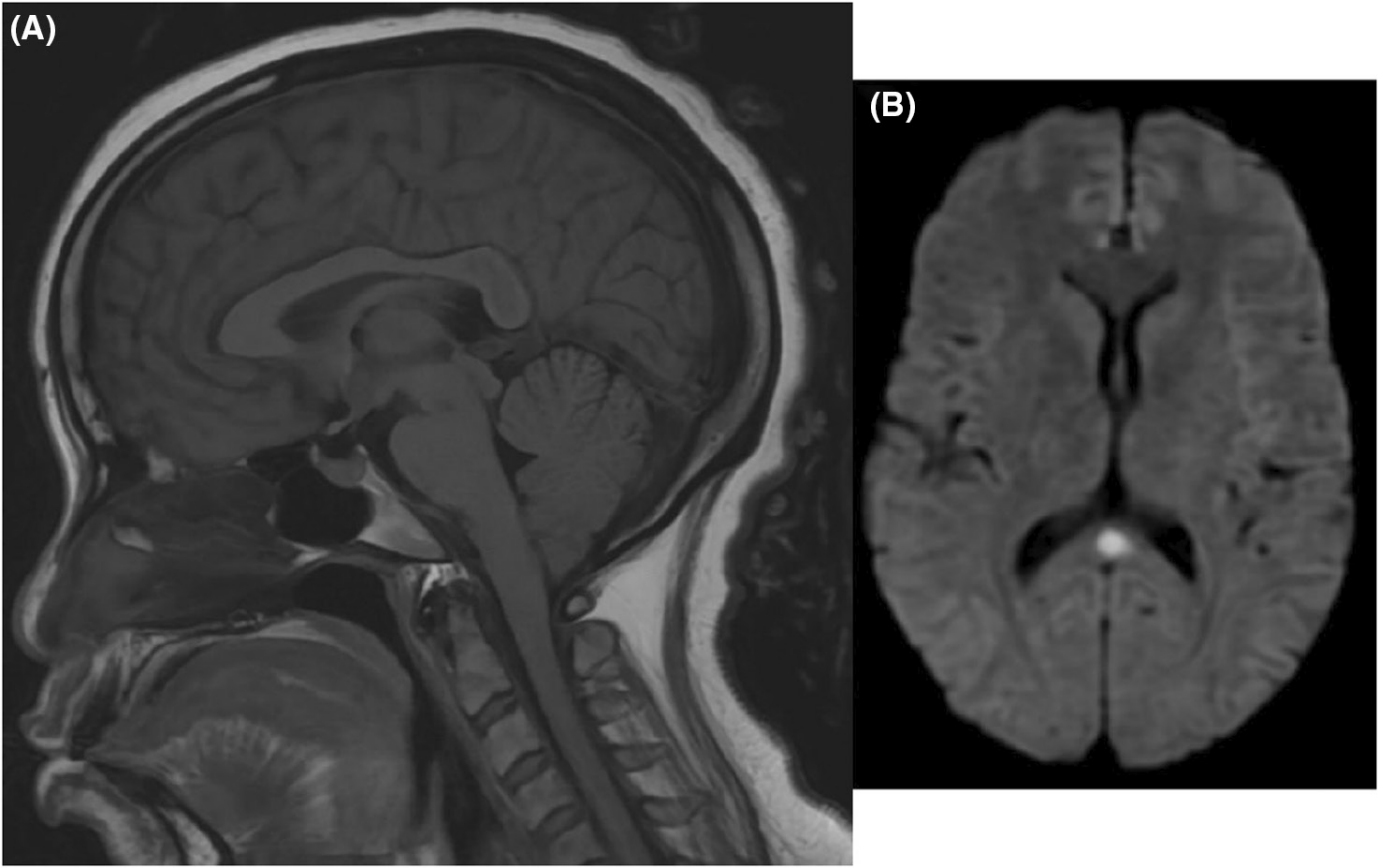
(A) Sagittal TI FLAIR reveals an enlarged and edematous corpus callosum, including the splenum. (B) Axial diffusion‐weighted imaging reveals a well‐defined ovoid lesion in the midline of the splenium of the corpus callosum with associated restricted diffusion.
